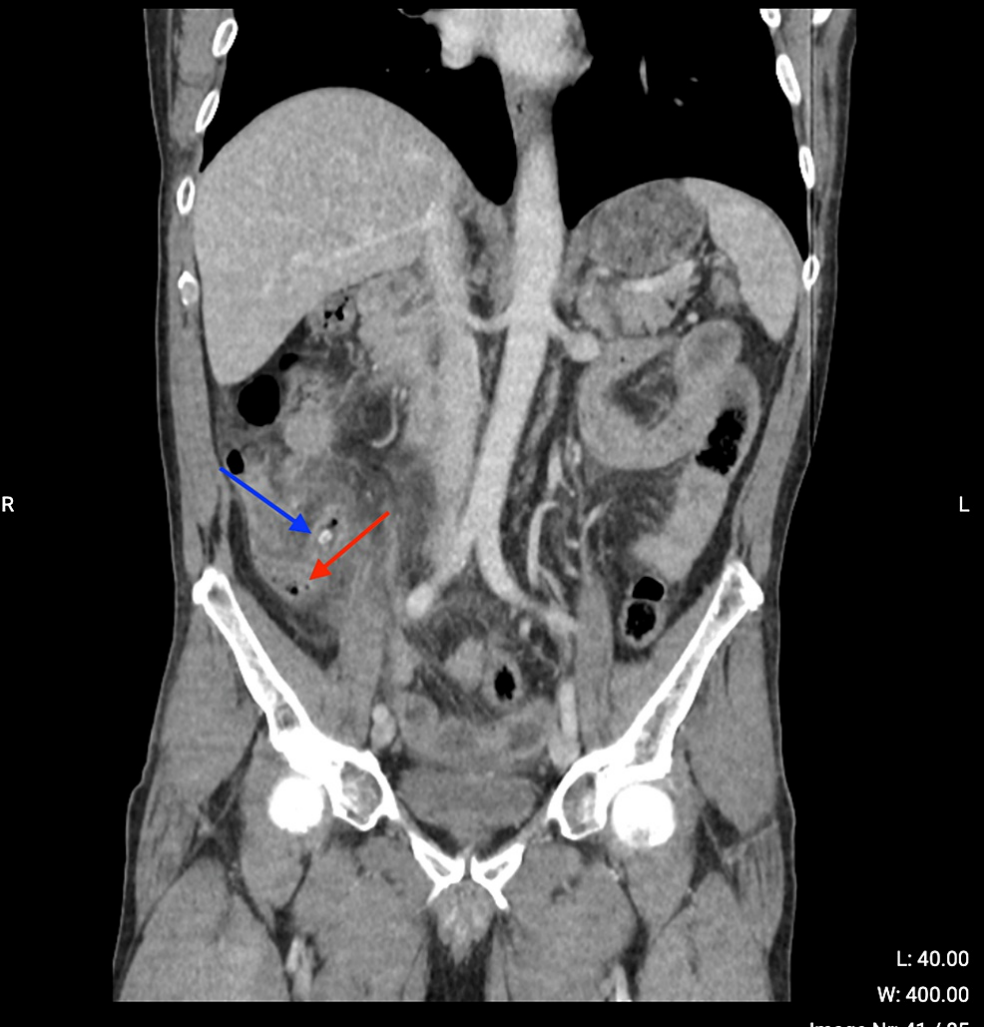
Abdominal CT scan. CT scan showing air alongside the appendix (red arrow) and stercolith (blue arrow).
